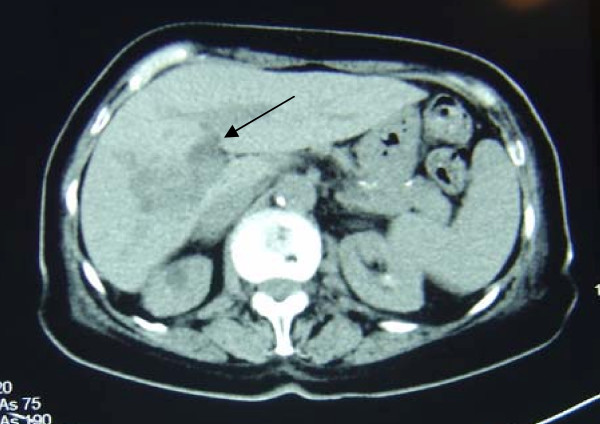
Abdominal CT scan showing area of low attenuation in right lobe of liver.
